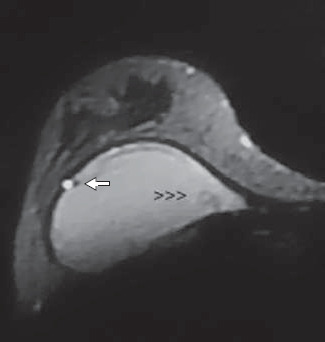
Intact silicone prosthesis with heavy gel bleeding identified at surgery. Axial fast spin echo magnetic resonance image of the right breast showing a small teardrop extending to the medial aspect of the implant (arrowheads) and a hyperintense water droplet *(arrow)* in conjunction with subcapsular lines.
